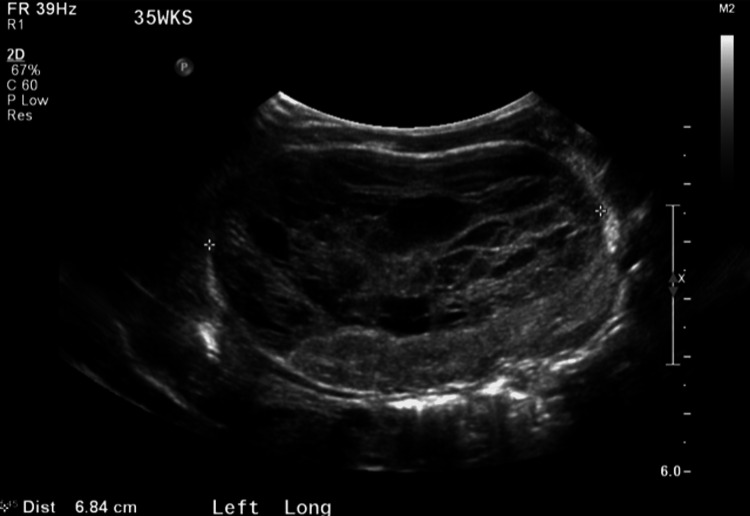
Ultrasound of the left kidney demonstrating septations within the perinephric fluid collection and compression of the kidney parenchyma.
